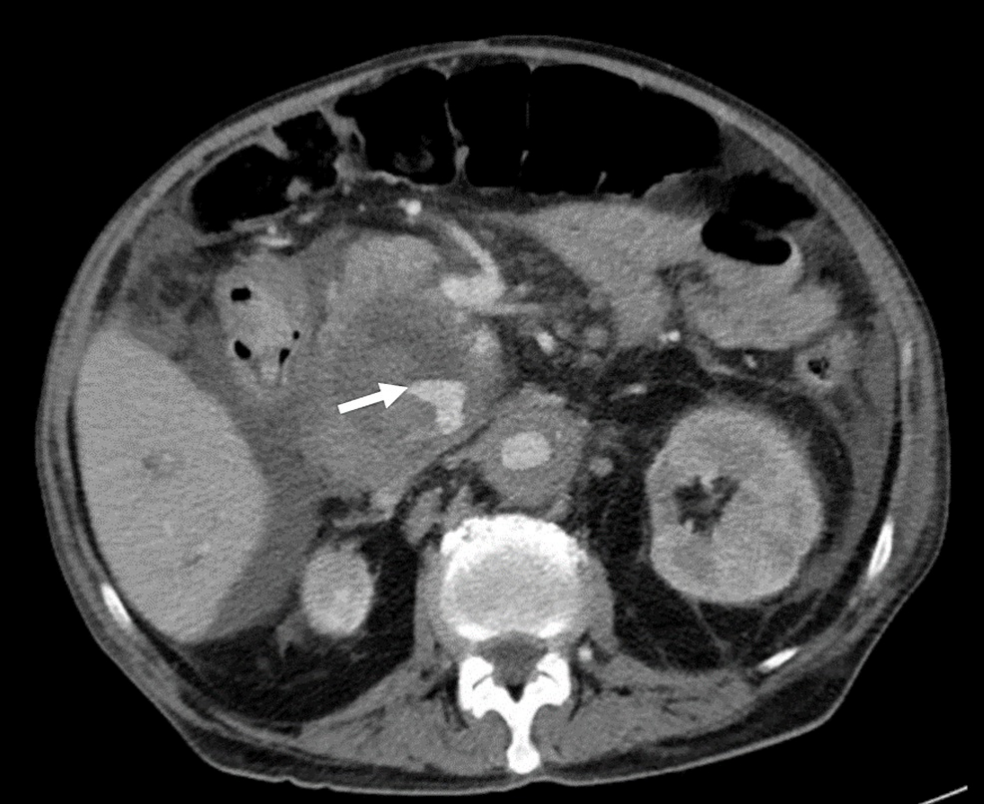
CT with angiogram of abdomen and pelvis (axial view). The white arrow points at a fluid level within the previously described mass represents active bleeding into a hematoma. The contrast enhancement of the fluid level suggests this is an active bleed.
